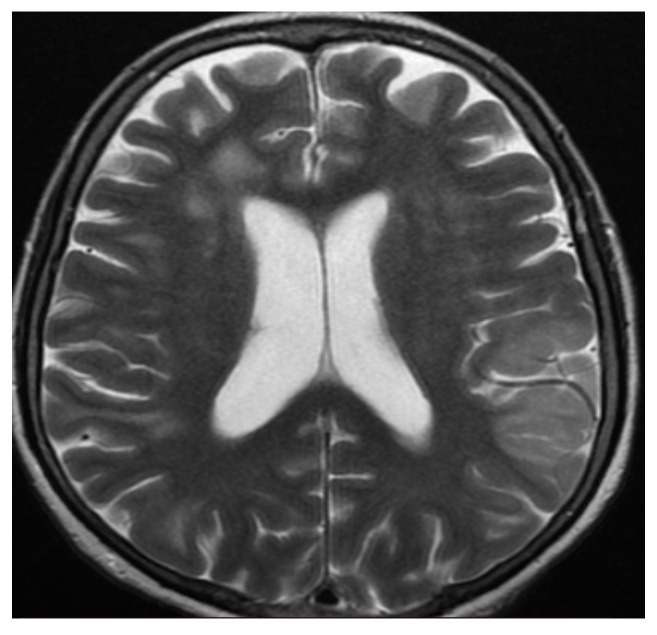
MRI axial T2-weighted sequence revealing bilateral diffuse confluent white matter hyperintensities in the frontal, parietal, and occipital lobes.
